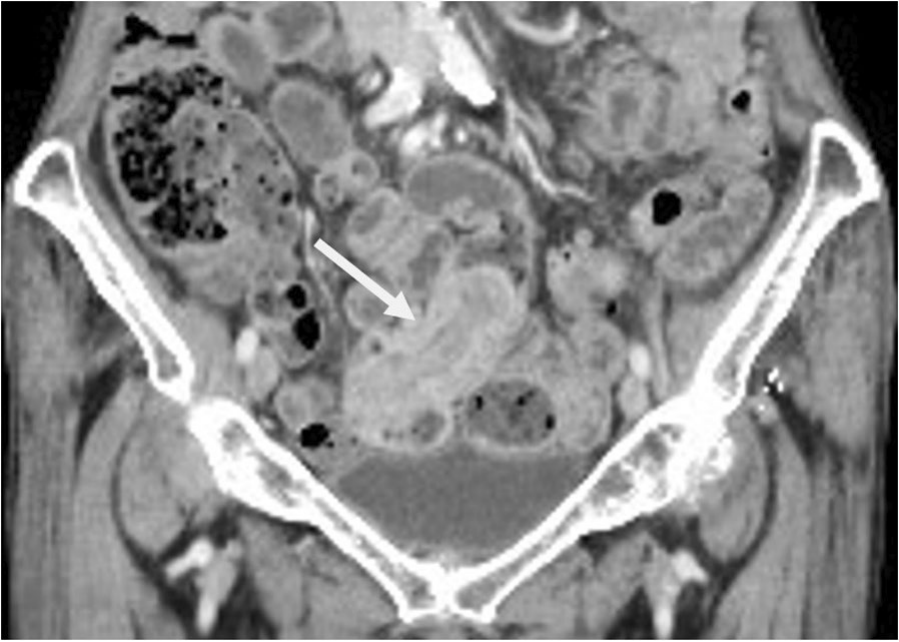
CT showed a tumorous lesion with contrast enhancement in the terminal ileum (arrow)
